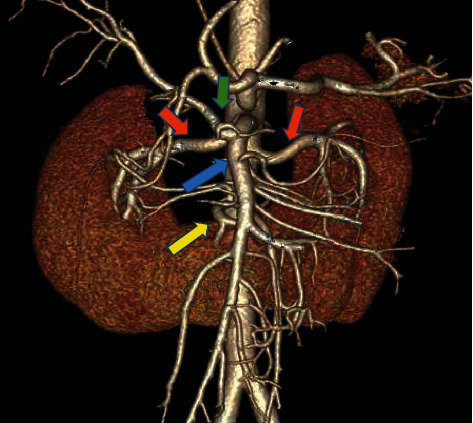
CT angiographic reconstruction of the vascular anatomy of the horseshoe kidney. Yellow arrow: the lower polar artery supplying the lower poles of both kidneys and the isthmus. Red arrows: the right and left main renal arteries. Blue arrow: superior mesenteric artery (SMA) originating from the aorta in very close proximity to the right renal artery. Green arrow: replaced right hepatic artery arising from the SMA.
